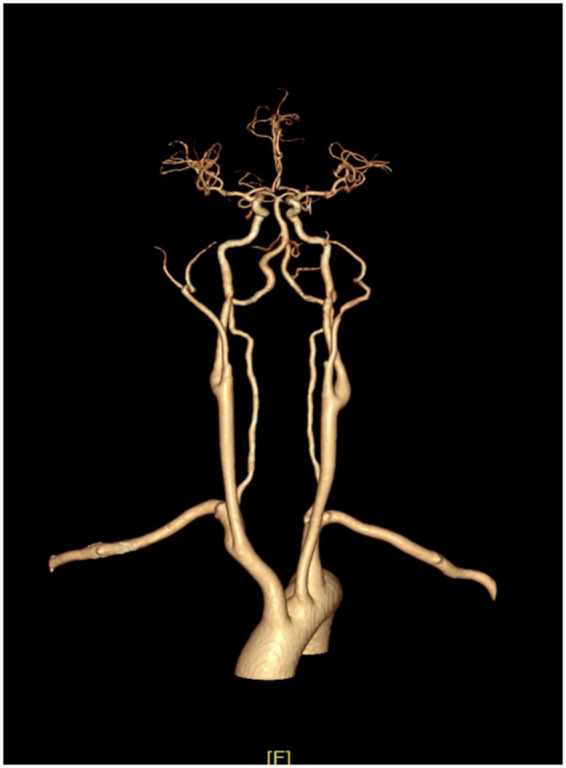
Head and neck CTA (May 27, 2025) depicting a congenitally smaller V4 segment of the left vertebral artery compared to the contralateral side with mild arteriosclerosis.
